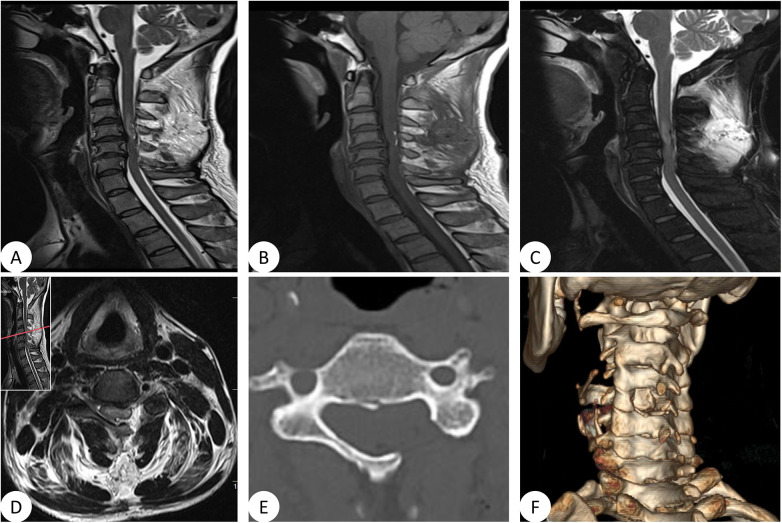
Magnetic resonance images of the cervical spine after surgery. (A–C) T1-weighted, T2-weighted, and fat-suppressed images demonstrated subtotal resection of the lipomatous lesion, with a small residual component remaining. (D) Axial T2-weighted images confirmed complete decompression. Computed tomography and three-dimensional reconstruction of the cervical spine after surgery. (E) Axial images showed that the left C4 lamina was removed. (F) Three-dimensional reconstruction showed that left C4 lamina was removed totally.
